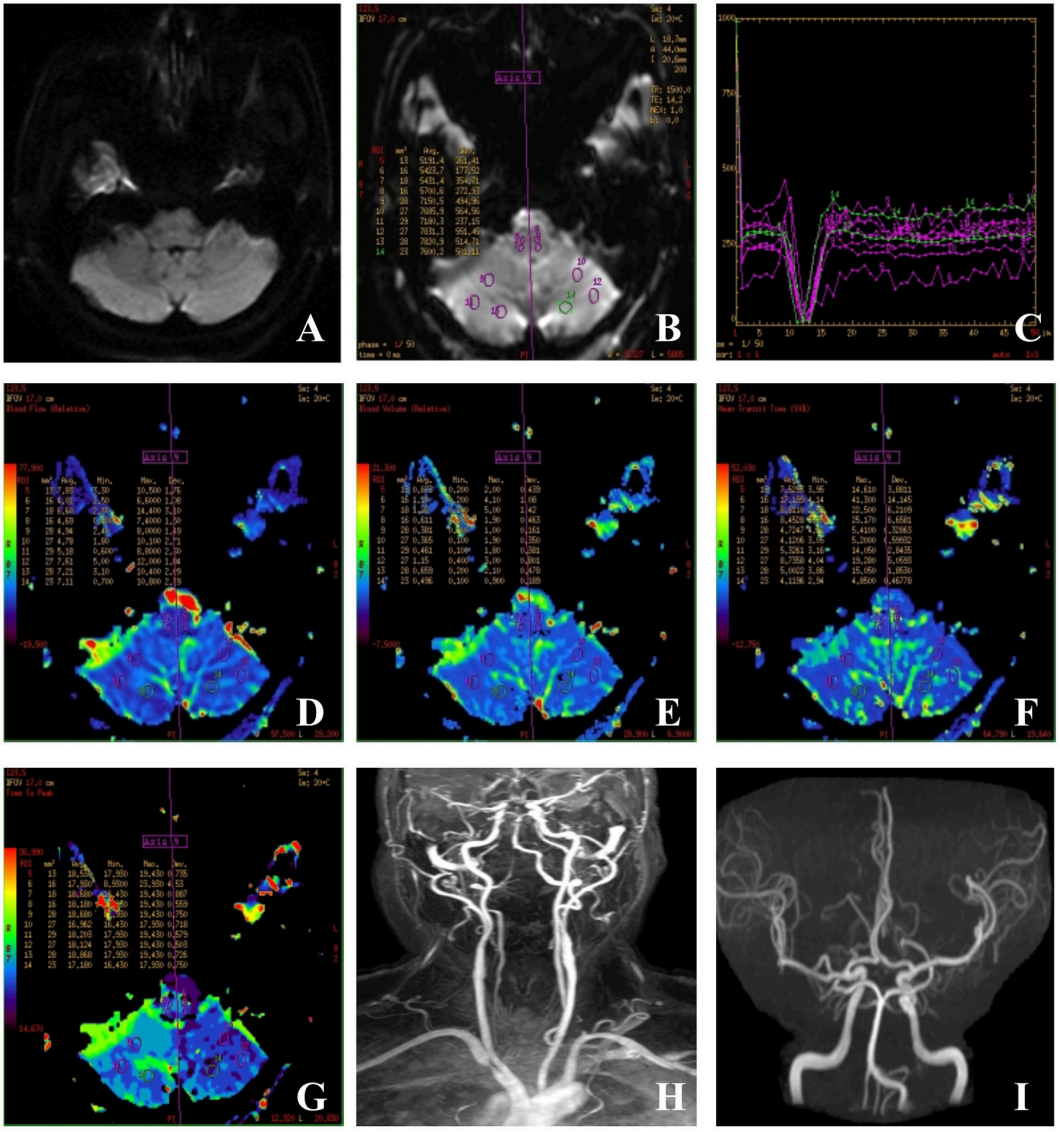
Images of a case of isolated vertigo suspected to be caused by hypodynamics of brain. (A) Sixty-one-year-old man had isolated vertigo on 4 April 2016 and performed with MRI, MRA, CEMRA, and PWI. (A) DWI-MRI was normal and showed no acute infarctions; (B) outlines of ROIs of bilateral cerebellum and medulla; (C) time-signal intensity curve; (D,E) cerebral blood flow (CBF) and cerebral blood volume: a similar color differences were not observed in the rCBF and rCBV panels. (F) Mean transit time (MTT): values of the right cerebellum varied from the left ones and are not obvious; (G) time to peak (TTP): TTP values obtained for the right cerebellum are longer than those obtained for the left cerebellum; (H,I) CEMRA and MRA showed the tortuous, dominant left vertebral artery, and severe stenosis at the beginning point.
